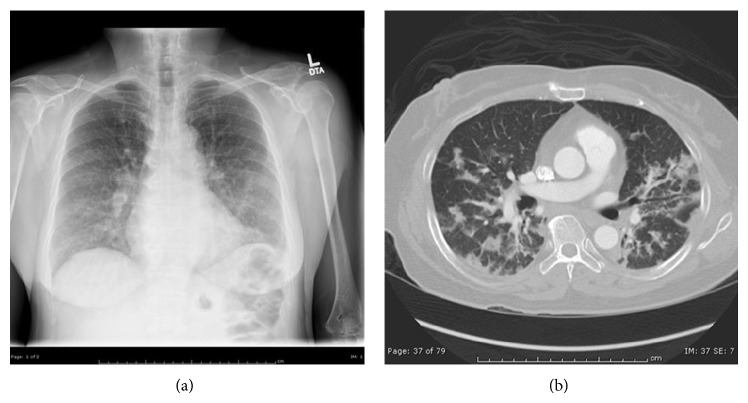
Lung imaging on admission. (a) Chest radiograph showing prominent interstitial lung markings. (b) CT pulmonary angiogram showing multifocal opacities and consolidation.
